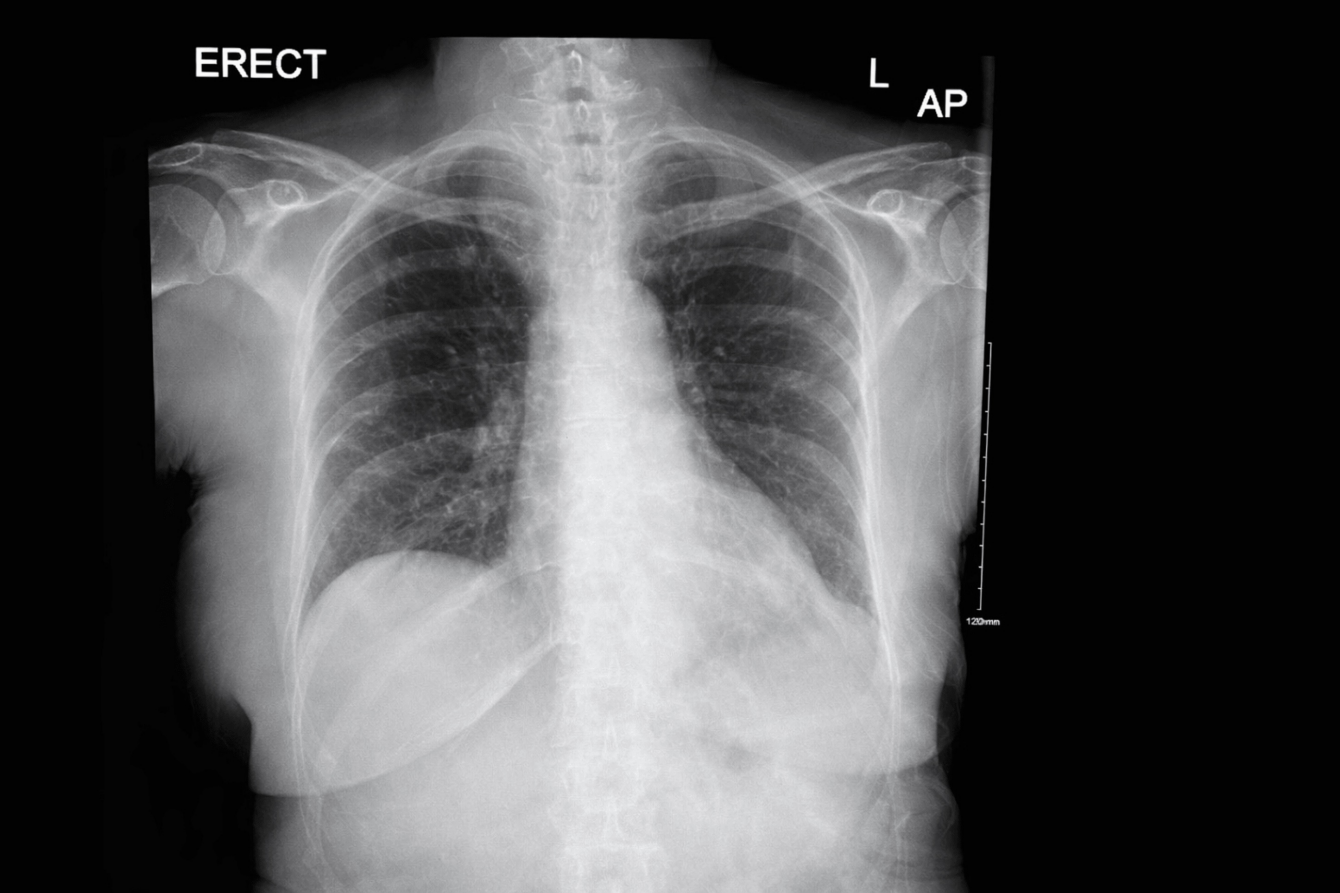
The Patient's Chest Radiograph Normal Cardiac and Mediastinal Contours With Clear Lung Fields. No Focal Consolidation, Pleural Effusion, Pneumothorax, or Acute Cardiopulmonary Abnormality Was Identified.
